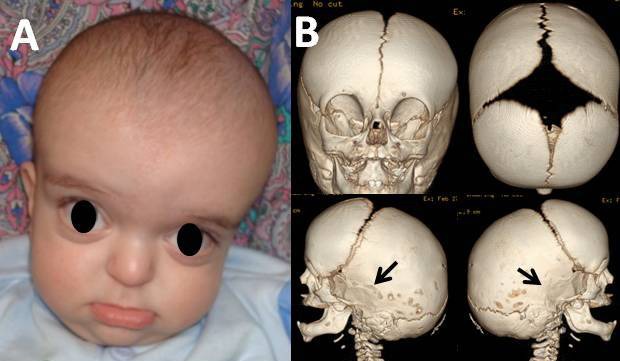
Patient picture at presentation (A). 3D reconstruction of computed tomography (CT) scan showed that the sutures of the cranial vault were widely open, while the squamosal suture, as well as parieto-mastoid suture, was fused bilaterally (arrows) (B).
